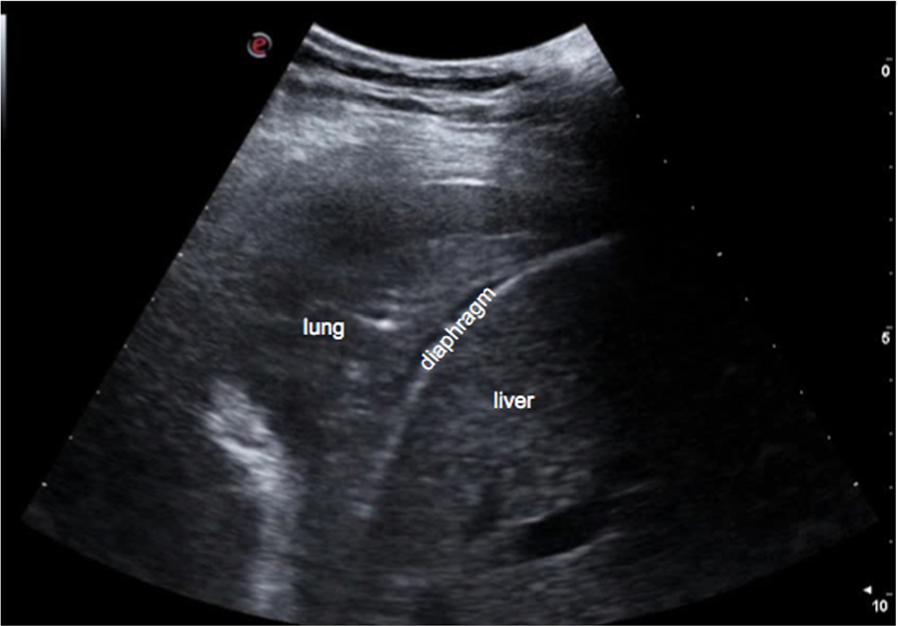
Sonographic appearance of a consolidated lung. The echo-texture of the lung becomes similar to the liver.
